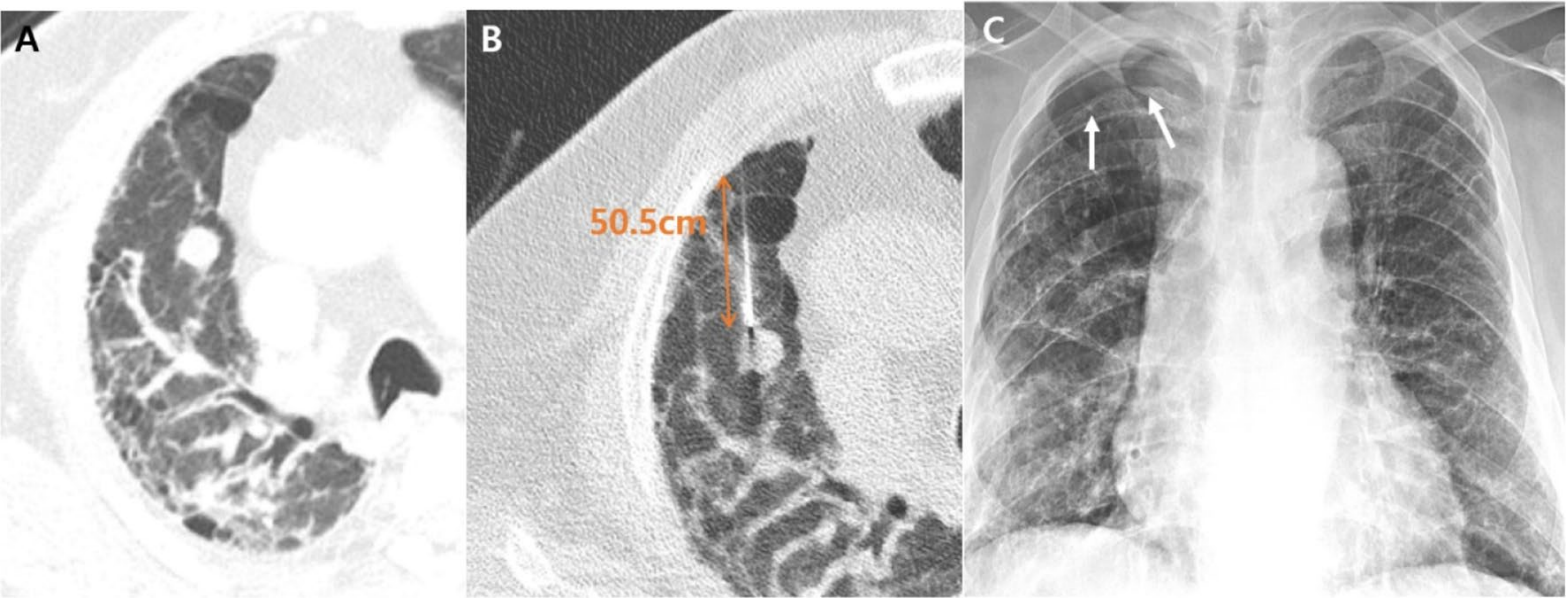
A 69-year-old male patient with idiopathic pulmonary fibrosis. The patient showed a 1.4-cm nodule in his right upper lobe (A). CT-guided percutaneous core needle biopsy (PCNB) was performed with the patient in the supine position. The length of the needle path (pleura to lesion) was 5.1 cm (B). Small cell carcinoma was confirmed through PCNB. He presented with pneumothorax after PCNB on follow-up radiography (C).
